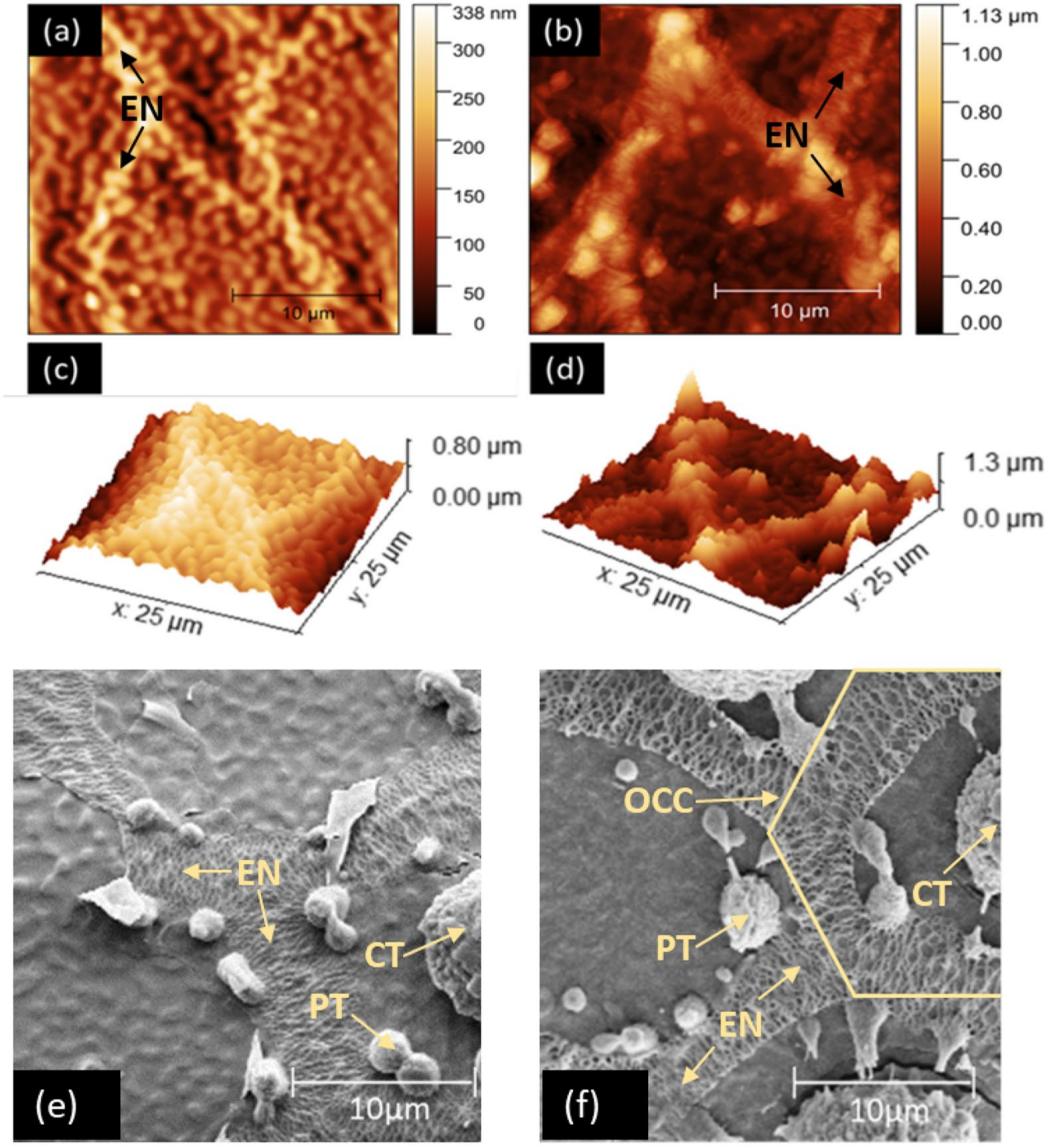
Surface topography of the mid-dorsal surfaces of JFW and JBW colony eggs by AFM and SEM. AFM images from G70 JFW eggs in (a) 2D and (c) 3D; G70 JBW eggs in (b) 2D and (d) 3D. SEM images of (e) G73 JFW and (f) G73 JBW eggs. CT-central tubercle; EN-exochorionic network; OCC-outer chorionic cell; PT-peripheral tubercle. Ten µm scale bars are shown.
